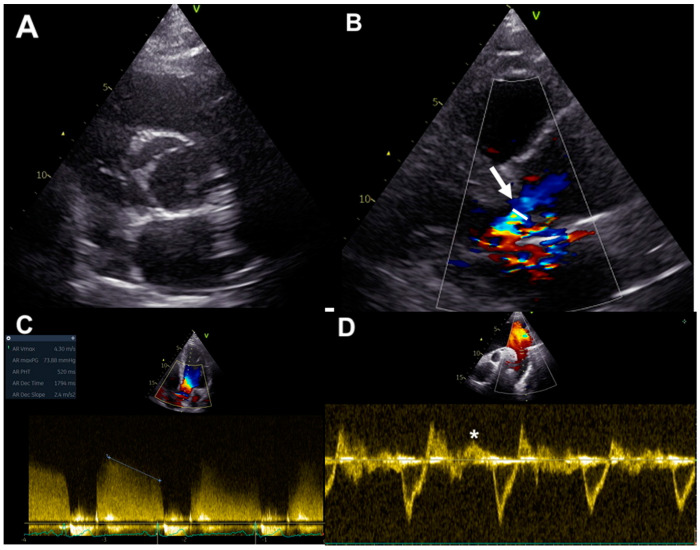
BAV assessment by echocardiography: (A) valve anatomy in parasternal short axis; (B) vena contracta width in parasternal long-axis (arrow); (C) pressure half-time measurement in continuous-wave Doppler; (D) assessment of the diastolic flow reversal velocity in the descending aorta by pulsed-wave Doppler (*).
